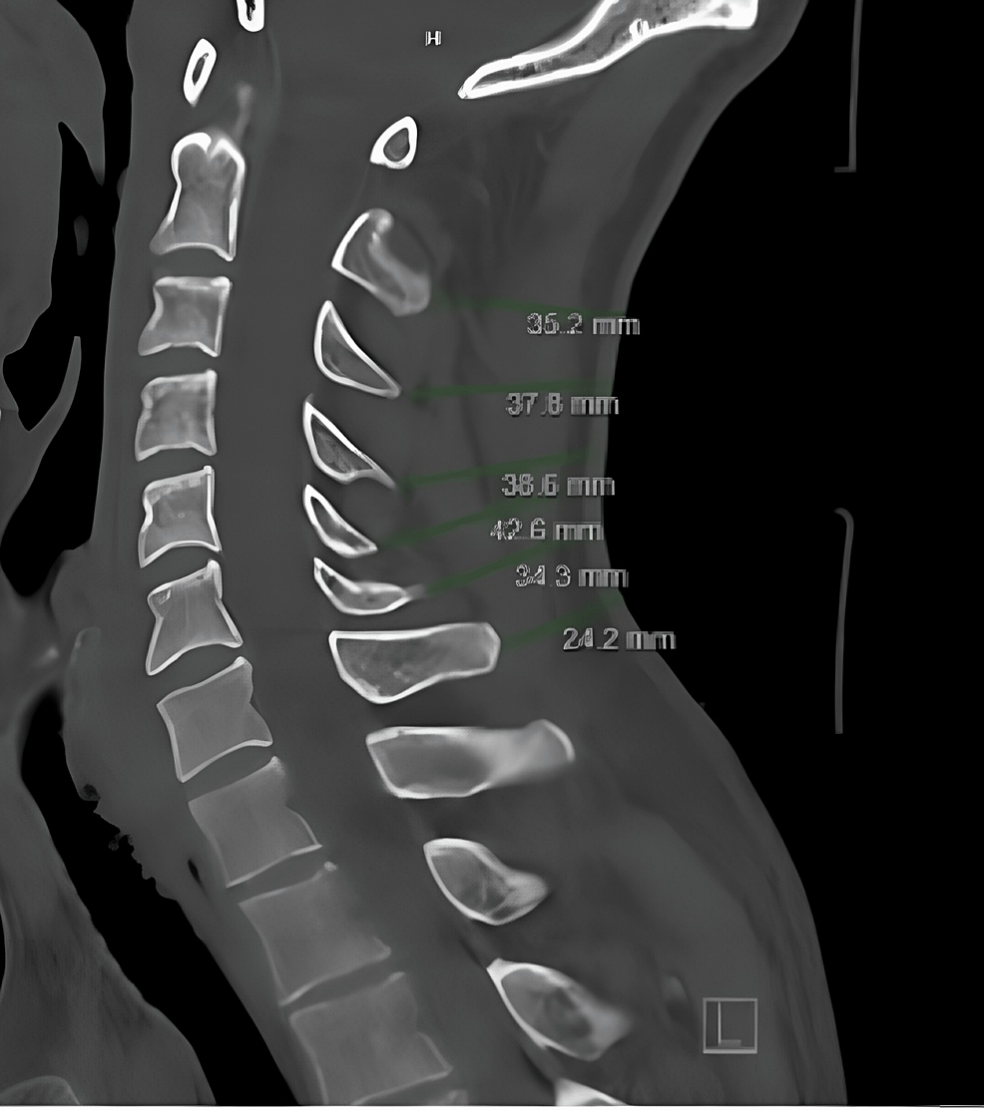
Representative sagittal cervical CT scan showing standard C2-C7 measurements
